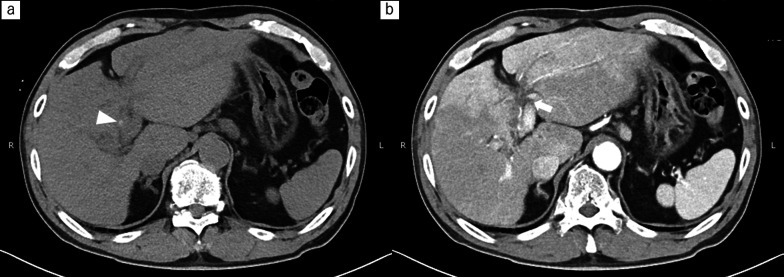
Computed tomography (CT) images of Case 2. a CT revealed intrahepatic lithiasis (arrowhead), and intrahepatic biliary dilation (arrow). b Early enhancement around the dilated hepatic duct suggested acute cholangitis.
